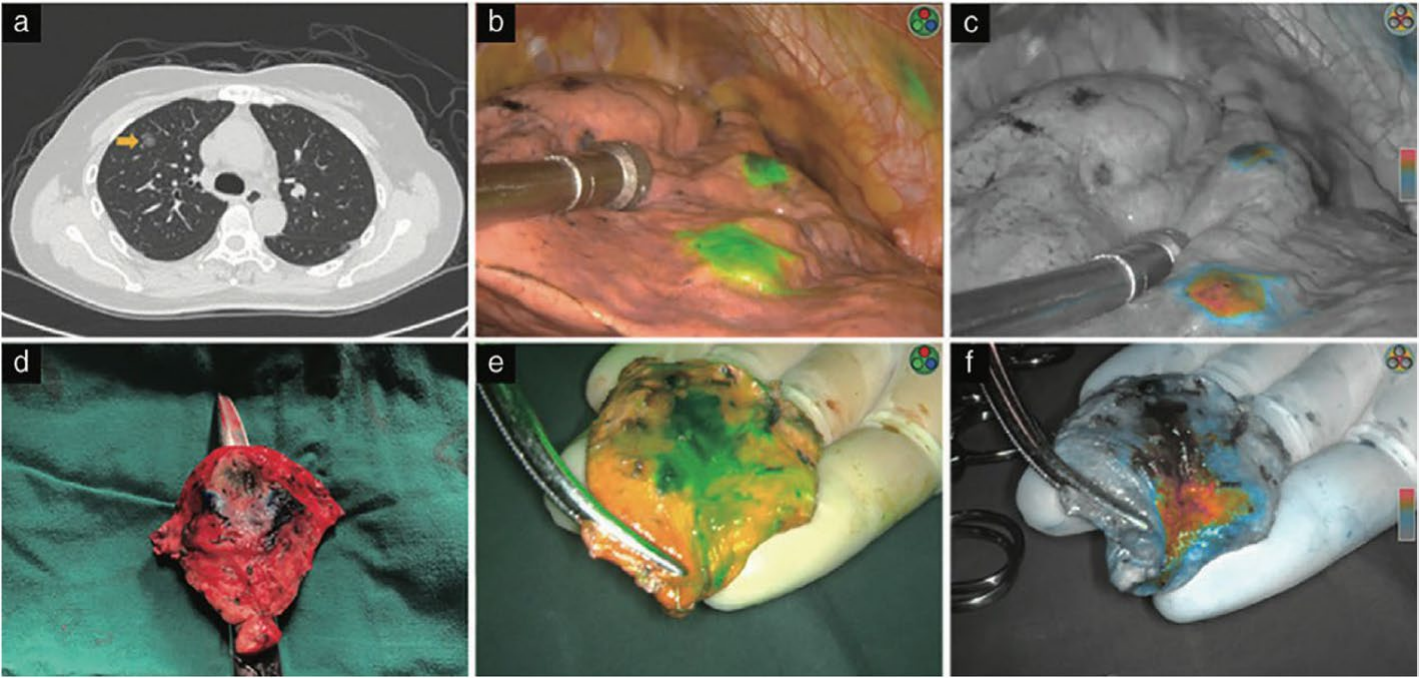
The CT-guided indocyanine green localization [5]
